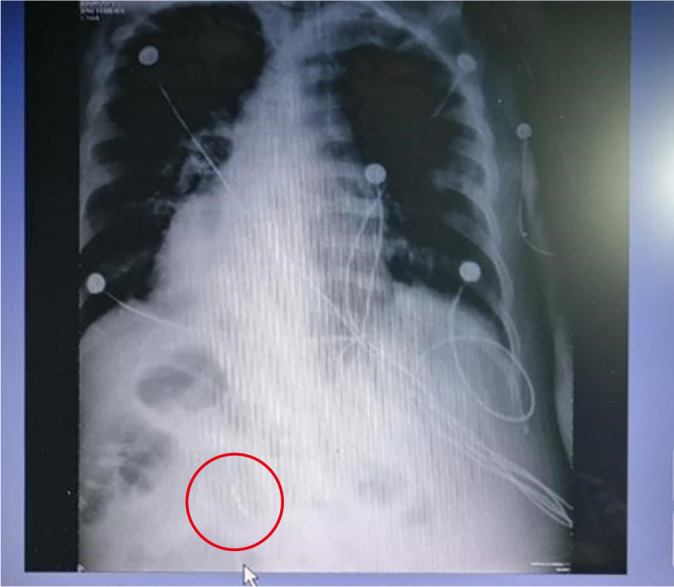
Radiograph following blind post-pyloric tube placement
